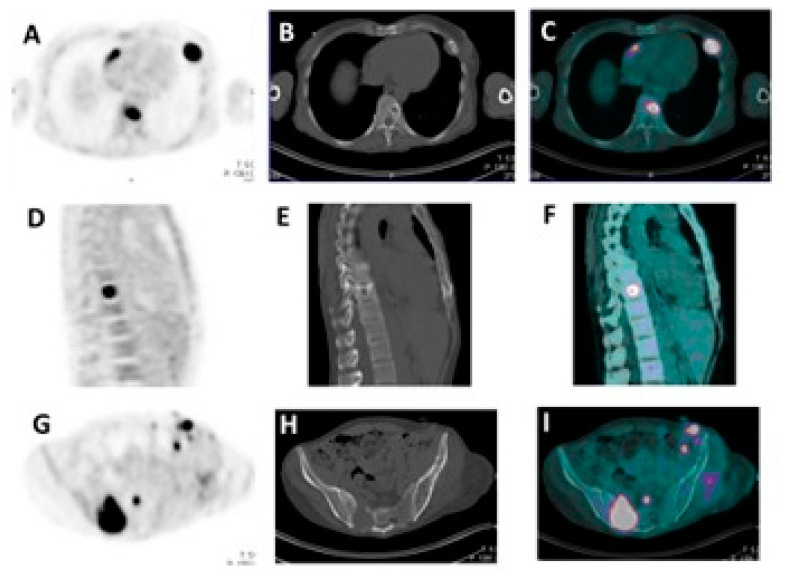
A representative example of hot spots with lytic lesions underlying (A,G) PET axial cut; (B,H) CT axial cut; (C,I) fused images axial; (D) PET sagittal cut; (E) CT sagittal cut; (F) fused images sagittal cut showing active disease in the spine (A–D), sacrum (G–I) and in the seventh rib on the left. (A–C). A pelvic subcutaneous lesion is also evident (G–I). In this case, IMPeTUs criteria would have been scored as BM4 (increased bone marrow uptake), F2 (three focal hot lesions) with DS4Sp (spinal) and ExP (extraspinal in the rib), EMsk (extramedullary skin).
